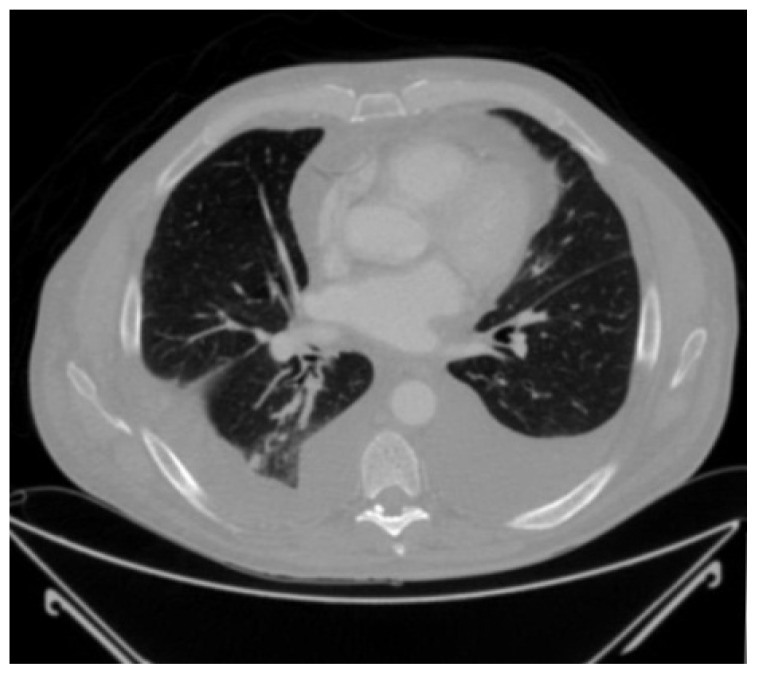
CT chest showing bilateral pleural effusions and clear lung parenchyma.
